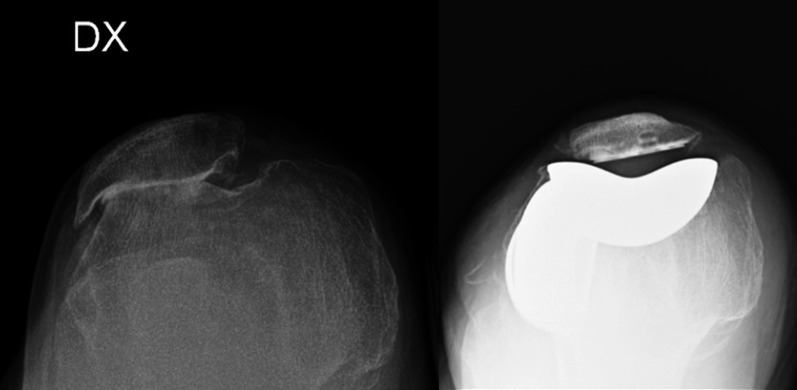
Axial right  knee radiographs showing a high degree of osteoarthritis of the patellofemoral joint, with abolition of the joint line treated with isolated patellofemoral arthroplasty
